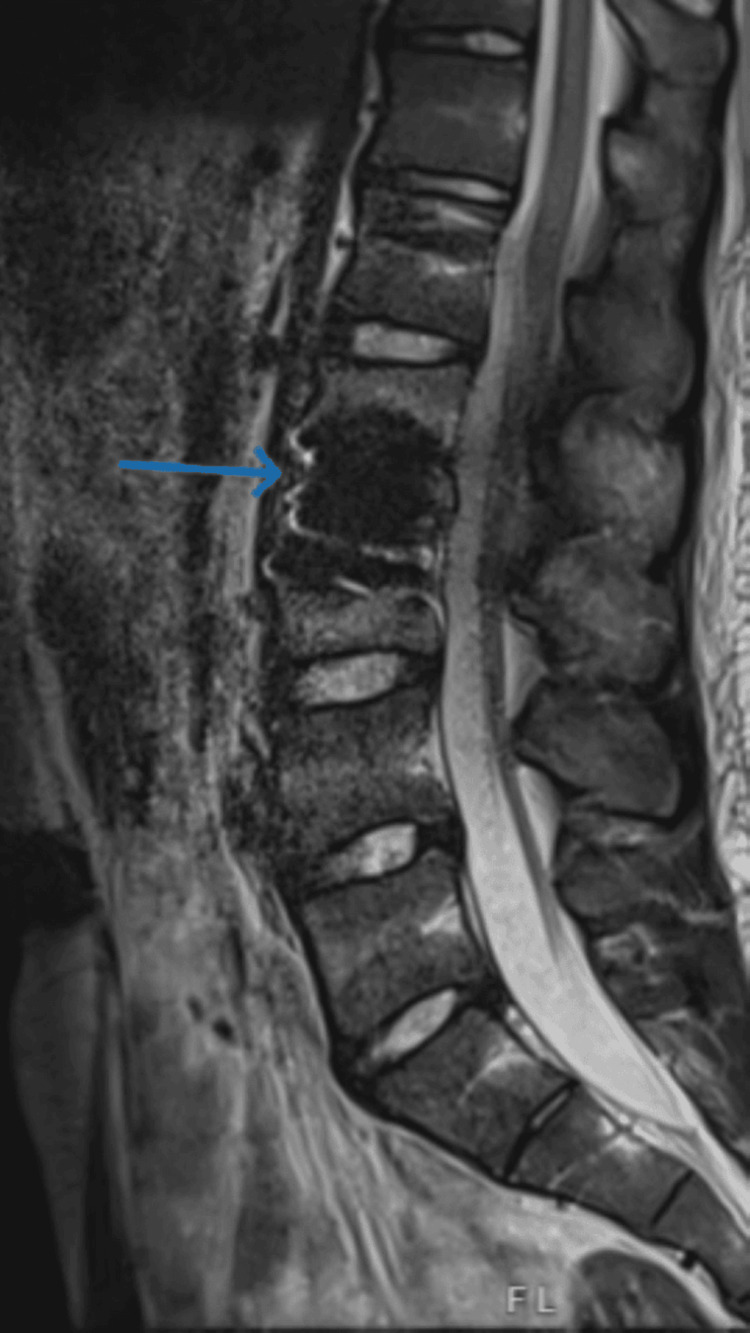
MRI of the lumbar spine with and without contrast showing worsening discitis osteomyelitis involving the entire L2-L3 vertebral bodies and intervertebral disc, with significant loss of disc height and an extension of infection and enhancement into bilateral psoas muscle without abscess. MRI: magnetic resonance imaging
